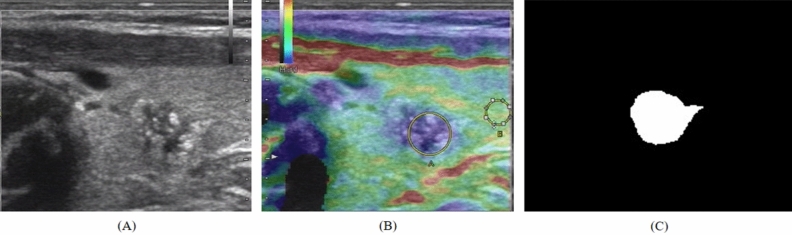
(A) Ultrasound conventional B-mode image of papillary thyroid carcinoma. (B) corresponding ultrasound elastography image, with the circle, labeled A indicating a lesion region and the circle labeled B indicating a reference area. (C) Corresponding image after region of interest (ROIs) segmentation step.
